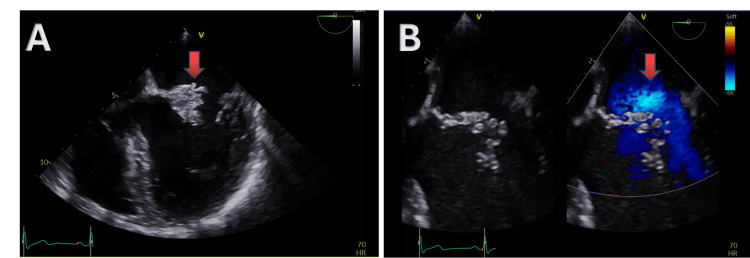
Transoesophageal echocardiogram (TOE) demonstrating mitral valve involvement. A: large vegetation on the anterior mitral valve leaflet (red arrow); B: color Doppler showing severe mitral regurgitation (red arrow).
